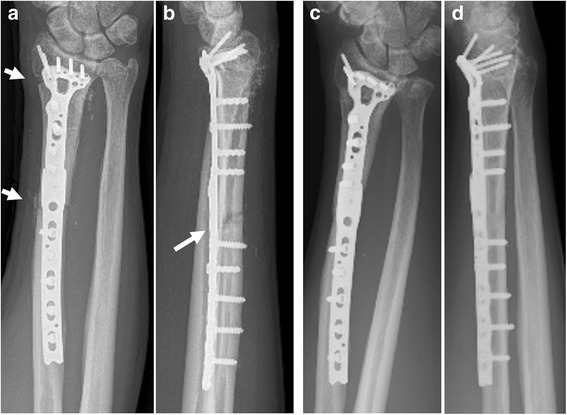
a, b Postoperative radiographs. Arrows indicate the osteotomy line. c, d Radiographs of the final follow-up. The bone was completely united and the osteotomy line was obscured
